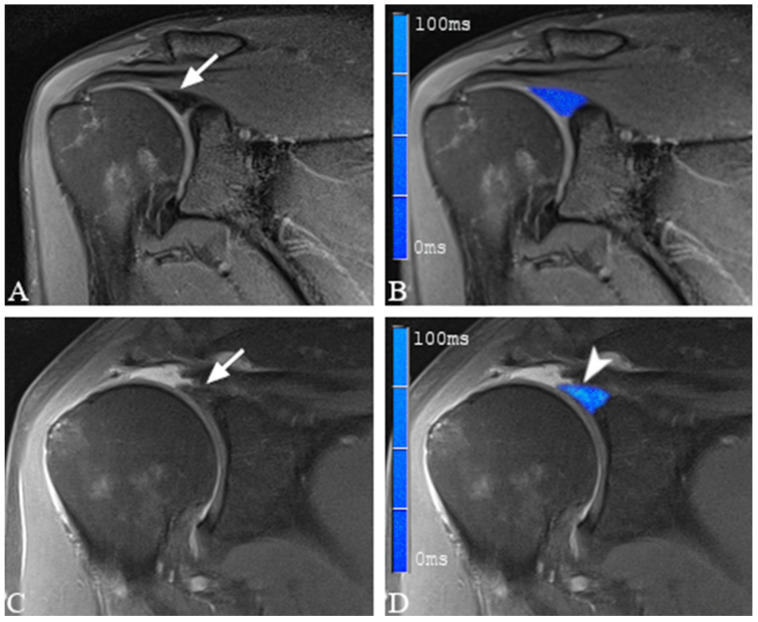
(A,C) show coronal proton density-weighted, fat-saturated magnetic resonance images of a 34-year-old woman with morphologically normal-appearing and arthroscopically proven healthy labrum (arrow in (A)), as well as fraying of the superior labrum in a 57-year-old man with arthroscopically proven SLAP lesion Type 1 (arrow in (C)). (B,D) show merged images of the proton density-weighted images and the corresponding color-coded T2 maps. Note the elevated average T2 mapping value of 53.9 ms in the damaged labral substance (arrowhead in (D)). The average T2 mapping value for the healthy labral substance was 21.3 ms (B).
